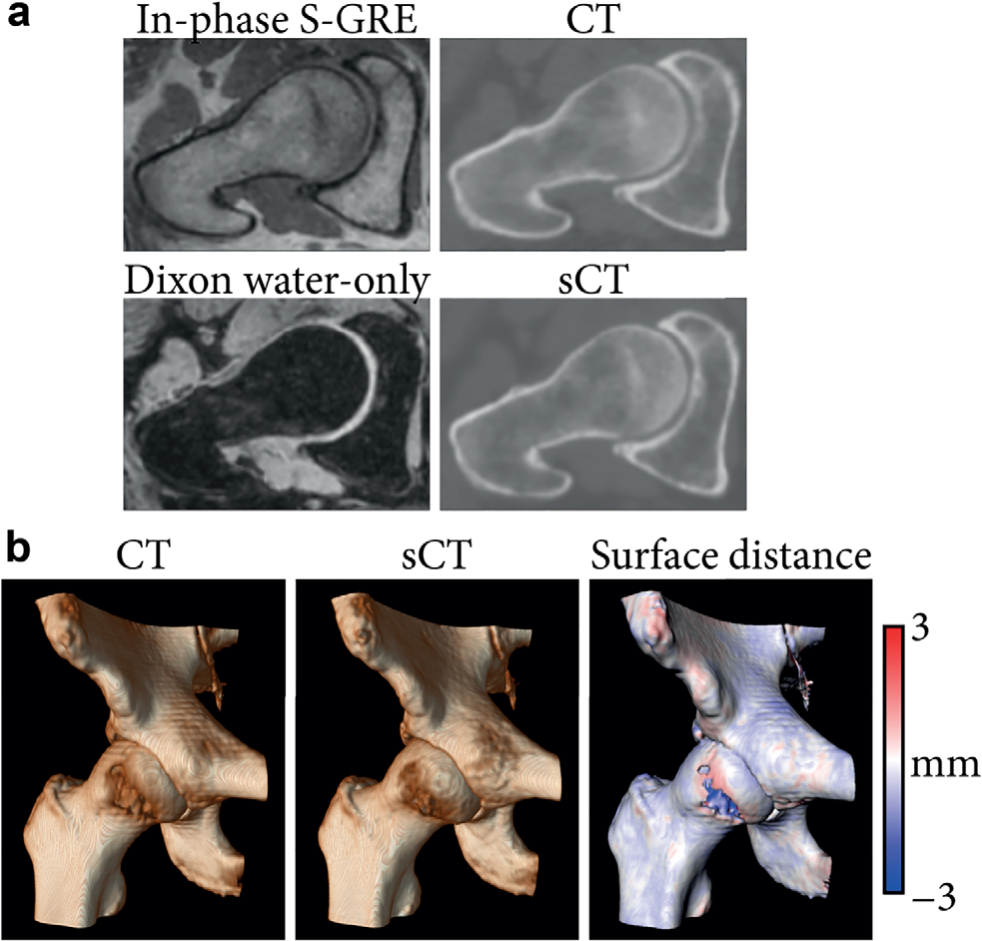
Magnetic resonance imaging (MRI)‐ and computed tomography (CT)‐based hip imaging. (a) Radial reformats of in‐phase radiofrequency spoiled gradient‐echo (in‐phase S‐GRE), Dixon reconstructed water‐only, CT, and synthetic CT (sCT) images. (b) Bone renderings obtained by applying a 150 Hounsfield unit threshold on CT and sCT images of a hip joint. Surface distance between the CT‐ and sCT‐based renderings was computed and mapped on the CT‐based rendering. Negative values indicate the CT is larger.
